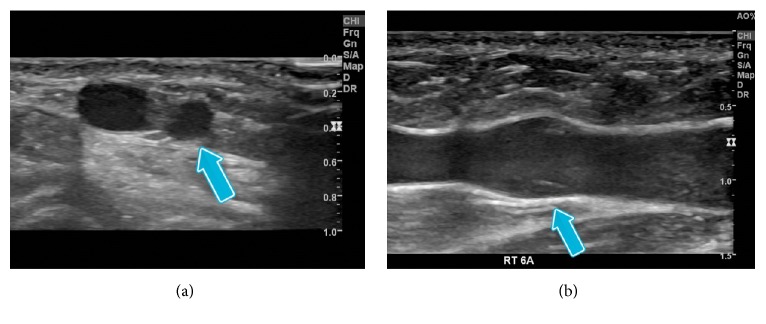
(a) Ultrasound image of branching vein (arrow) joining studied vein and (b) ultrasound image of valve showing two linear and symmetrical valve leaflets in dilated vein sinus (arrow).
